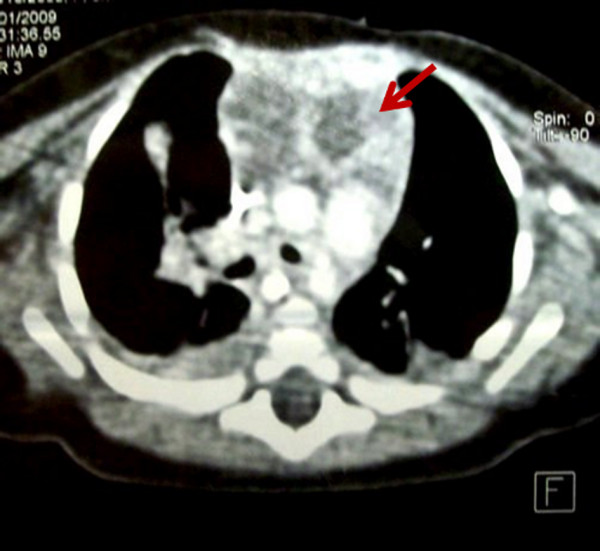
Chest computed tomography scan showing an anterior mediastinal multiseptated mass with areas of lower density (arrow).
